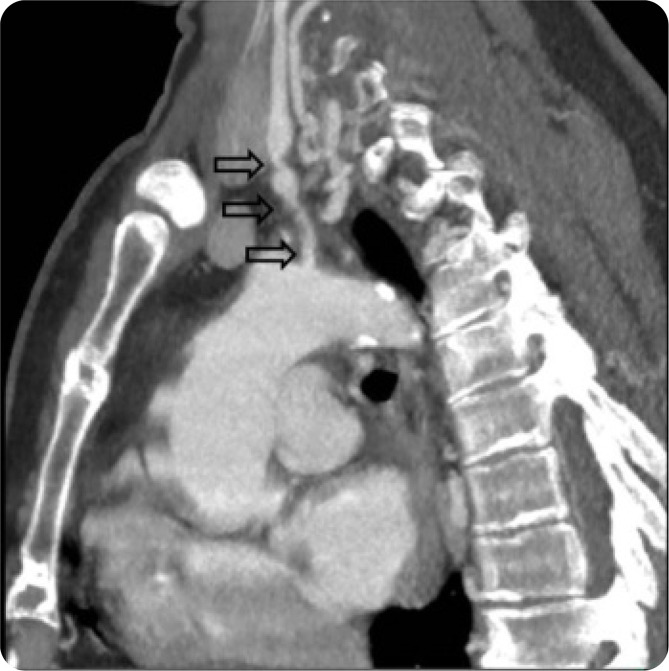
Sagittal CTA chest reformat focusing on the left common carotid artery showing multifocal stenosis (black arrows).
